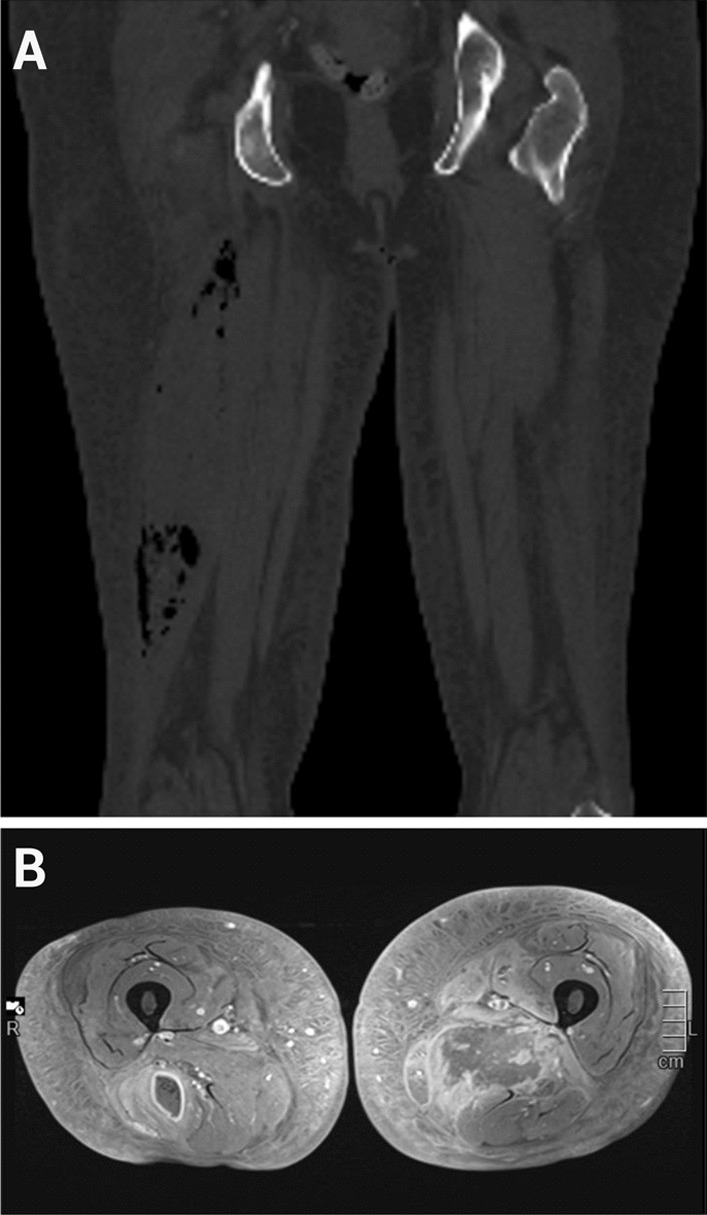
A Computed tomography of the thighs without contrast enhancement showing emphysematous myositis of the hamstring muscle in the right thigh. B Intravenous gadolinium-enhanced MRI scan of both thighs showing the emphysematous myositis and abscess with an enhancing ring and gas bubbles in the right biceps femoris muscle, and diabetic myonecrosis in the left adductor magnus muscle, with unenhancing intramuscular necrosis surrounded by enhancing peripheral reactive muscle edema
